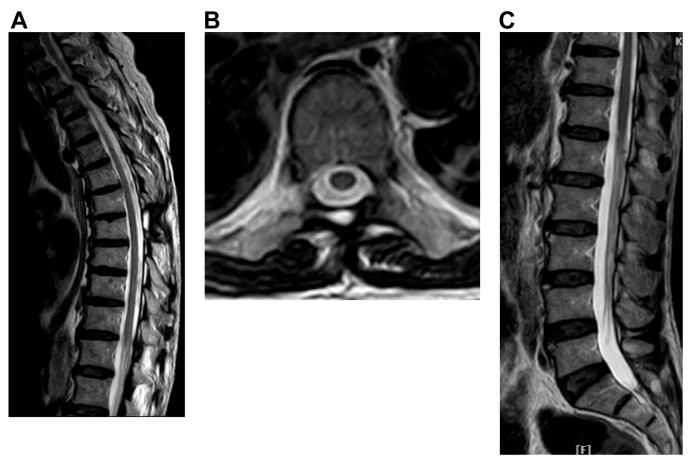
Post-op MRI 4 months after induction of chemotherapy. (A) T2-weighted sagittal image of thoracic spine showing no spinal cord compression by the tumor. (B) T2-weighted axial image of the thoracic region at the T8 level showing full expansion of the dural sac without tumor compression. (C) T2-weighted sagittal images of the cauda equina showing reappearance of CSF signals in the dural sac.
